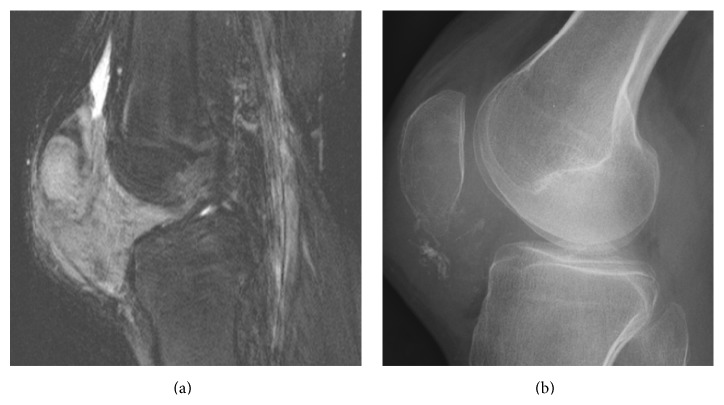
17-year-old boy with a large sarcoma in Hoffa's fat pad (case #S4). (a) Sagittal fat-saturated T2-weighted MR image shows the tumor growing extra-articularly and invading the patella. (b) Lateral radiograph shows intratumoral calcifications.
